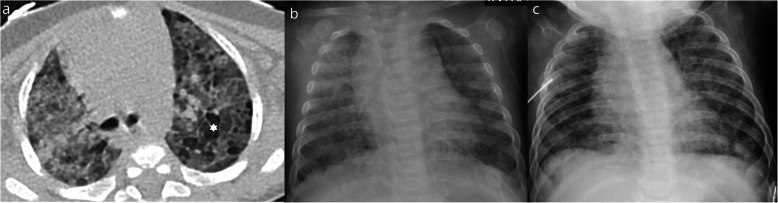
Indeterminate features. Chest CT of a 4-month-old infant on the third day of symptoms (a) shows diffuse cystic lucencies throughout both lungs (*), compatible with known bronchopulmonary dysplasia. CT also shows superimposed diffuse ground-glass opacities which could be attributable to the confirmed COVID-19. CXR on the same day as CT (b) and 6 days later (c) shows improvement of the diffuse ground-glass opacities
